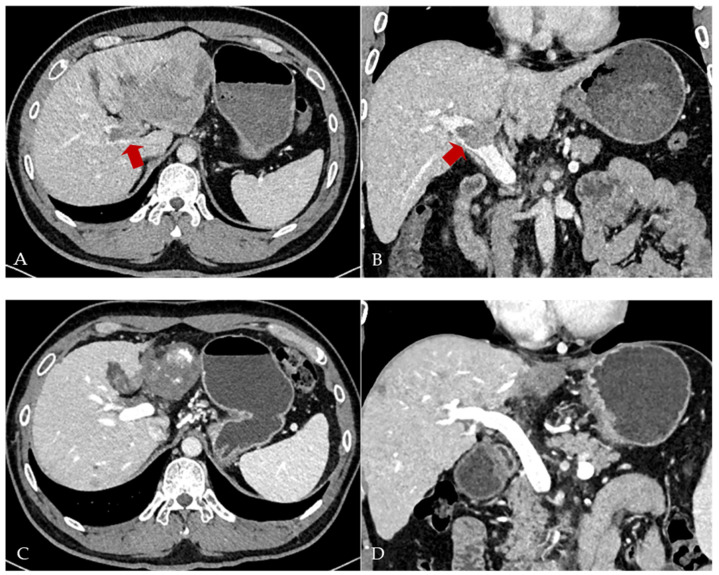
A 48-year-old man with hepatocellular carcinoma and major portal vein tumour thrombosis (PVTT) underwent combination therapy consisting of lenvatinib, transarterial chemoembolisation, and hepatic arterial infusion chemotherapy (Len-TACE-HAIC). (A,B) Pretreatment contrast-enhanced computed tomography (CT) revealed a 9.6 cm intrahepatic mass in the left lobe with major PVTT (arrow). (C,D) Follow-up contrast-enhanced CT performed 6 months after initial Len-TACE-HAIC demonstrated remarkable intrahepatic tumour shrinkage without enhancement and disappearance of major PVTT with patency of the main portal trunk.
